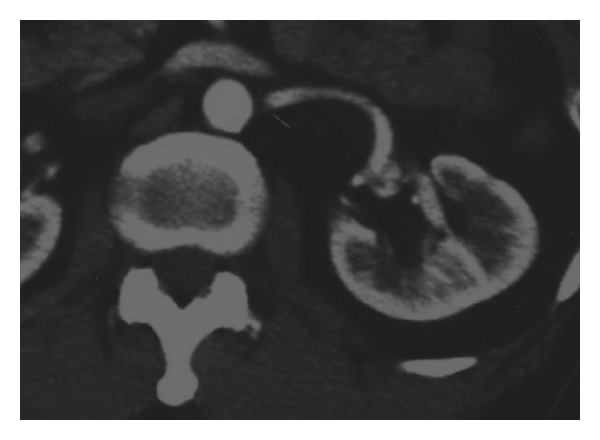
CT scan reveals retroperitoneal lipoma around the left renal pedicle, 30 mm in diameter, causing a curving of the left renal artery and dilatation of the left renal vein.
